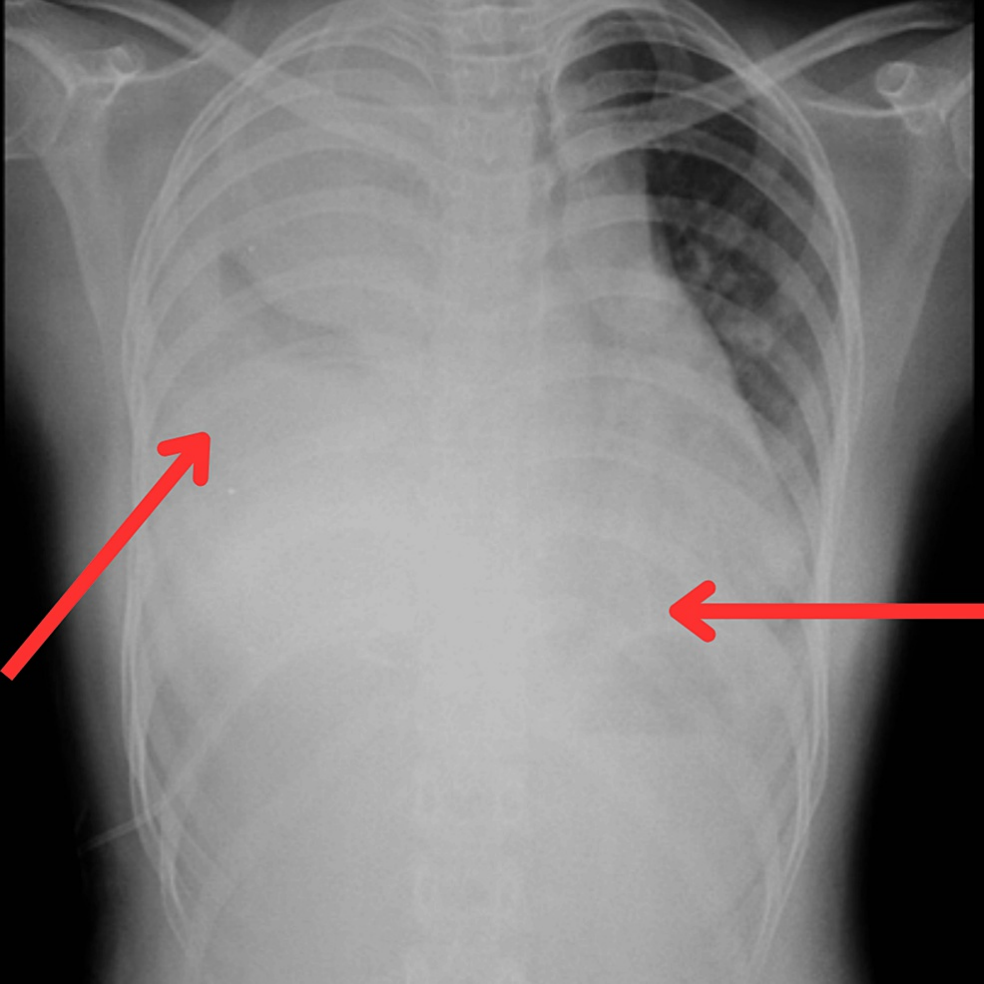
Chest X-ray (posteroanterior view) The arrow shows homogeneous opacity in the upper and lower zone of the right lung and the lower zone of the left lung along with the obliteration of both side costophrenic and cardiophrenic angles.
